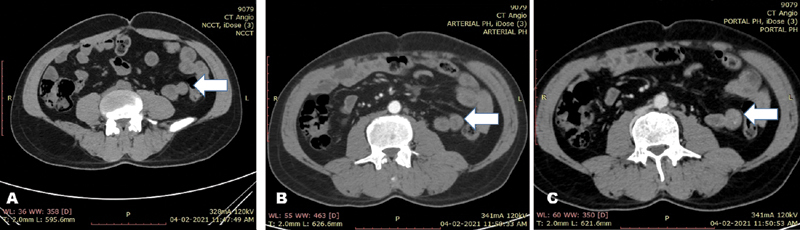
Vascular malformations (lesions filling in portal venous phase) in triple phase computed tomography scan (small bowel [
